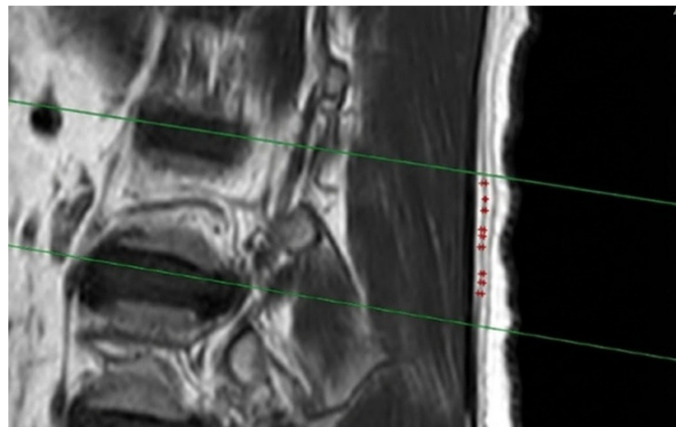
MRI assessment of TLF thickness in the sagittal plane at the L3 level. The upper and lower green lines mark the cranio-caudal boundaries of the L3 segment (above the superior endplate and below the inferior endplate). The red markers/short red lines indicate individual caliper measurements.
